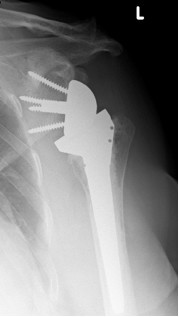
Antero-posterior radiograph of a 75 year-old female patient ´ s left shoulder with an implanted inverted total shoulder prosthesis Delta at 32 months of follow-up. Radiological analysis reveals "grade 4 = erosion over the inferior screw with extension under the base plate" of infraglenoidal scapular notching according to Nerot.
